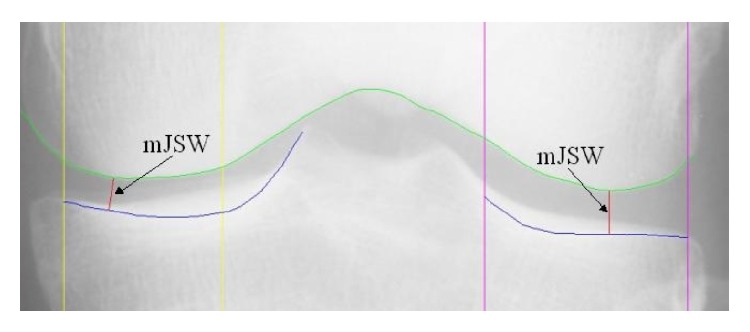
Output of the software on a digital knee radiograph. mJSW is the minimum JSW in a compartment. We provide mJSW for the medial compartment only (image from OAI).
